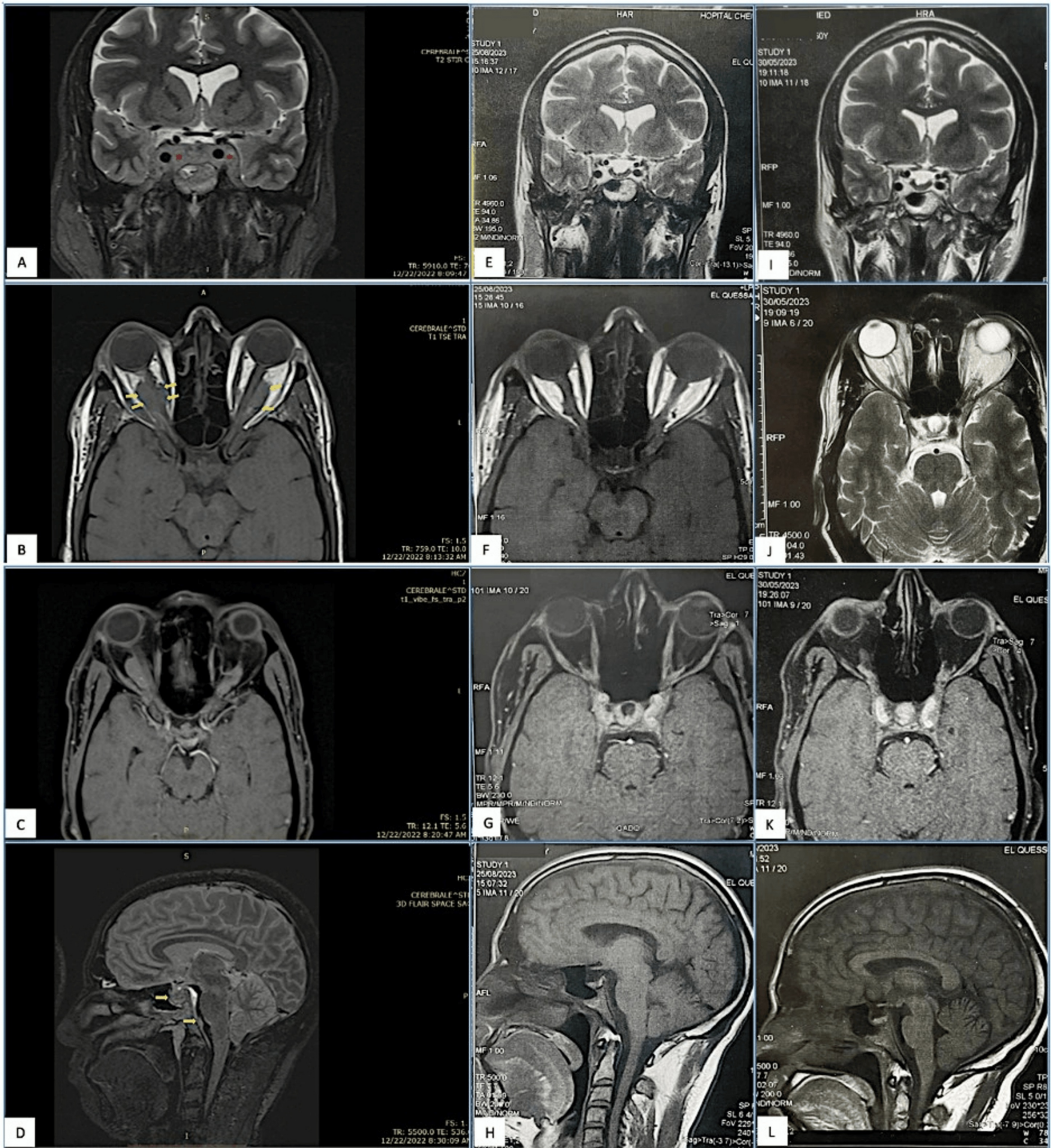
Orbital-cerebral brain MRI. AT initial evaluation (A-D): Infiltrating tumor process in the sellar region, extending into the cavernous sinuses (A). This process invades both orbital pyramids through the superior orbital fissure and encompasses the intracranial and posterior intraorbital optic nerves (yellow arrowhead B). In the sellar region, the pituitary parenchyma is surrounded on both sides by this process (C), which partially fills the optochiasmatic cistern, extending posteriorly toward the prepontine cistern (yellow arrowhead D). Screening after four cycles of chemotherapy (E-H) and after six cycles of chemotherapy (I-L). MRI, magnetic resonance imaging
